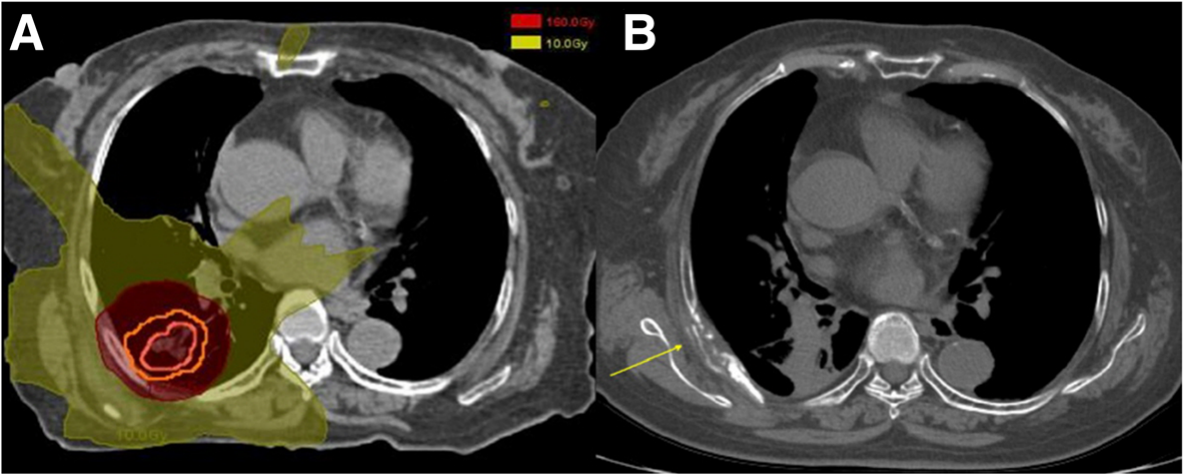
Example of radiation induced rib fracture and dose distribution. A 74-year-old man treated for adenocarcinoma in *right lower lobe* developed rib fracture after 37 months. The prescribed dose was 66 Gy in 3 fractions. a Dose distribution in the transverse plane corresponding to the site of rib fracture. *Red area* represents the *isodose line* of 160 Gy in EQD2. b Rib fracture detected on follow-up CT (*arrow*)
